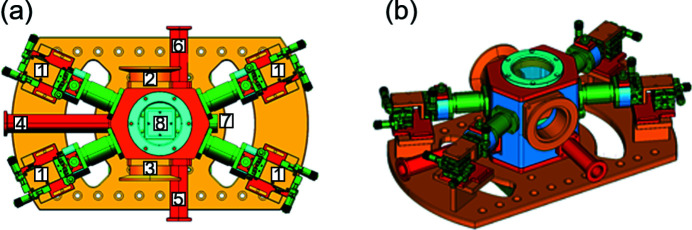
Conceptual design of the miniature X-ray probe station. (*a*) Top view and (*b*) perspective view. It mainly consists of a miniature vacuum chamber and motion stages for the probe holder.
